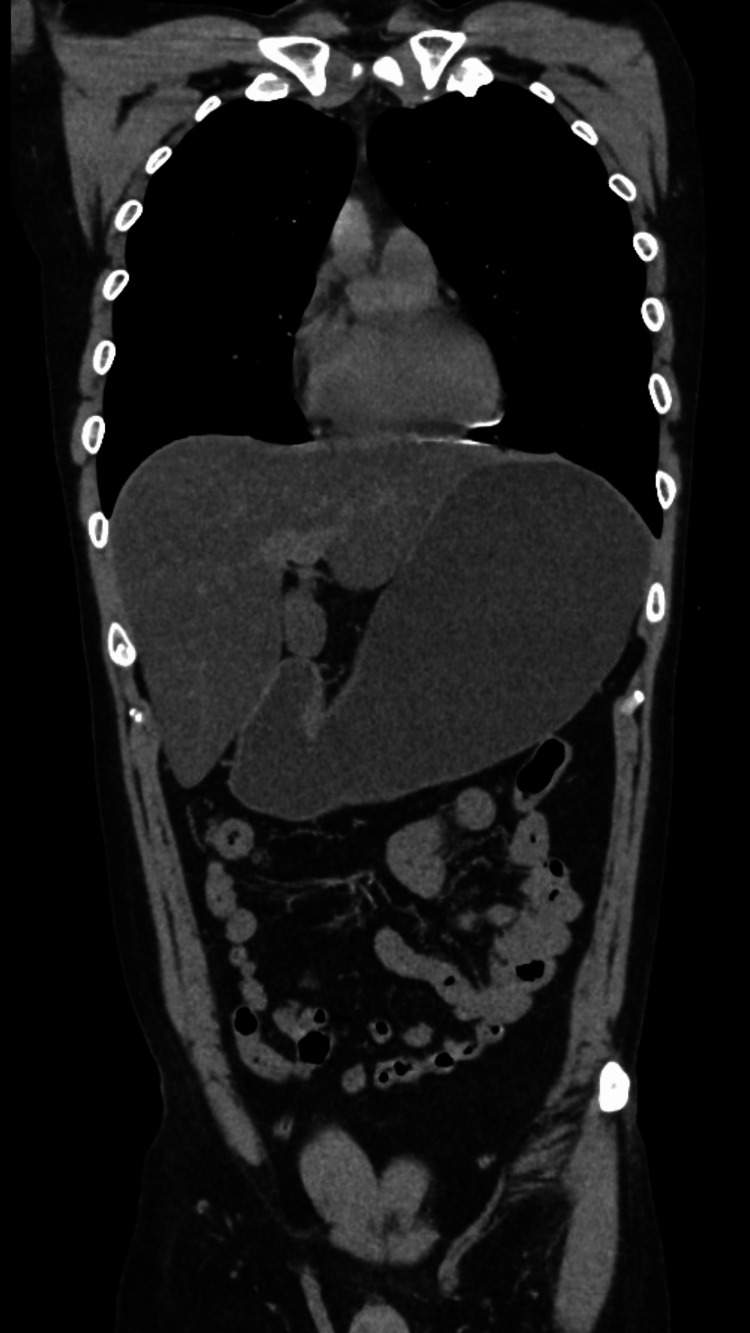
CT scan taken upon emergency admission The patient reported approximately 30 episodes of vomiting prior to the imaging. The scan reveals marked gastric distension with no evident abnormalities distal to the small intestine.
